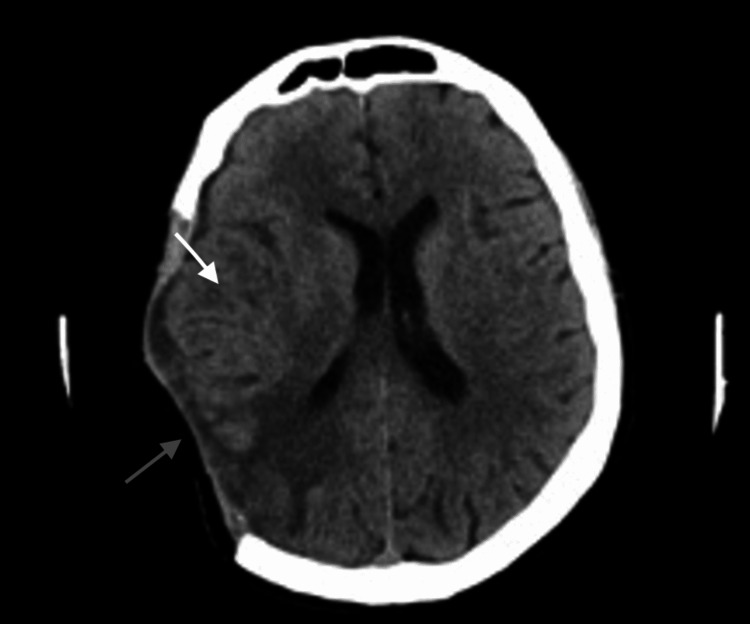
Computed tomography angiogram scan of the head showing postoperative changes (gray arrow) with right hemispheric edema similar to prior studies (white arrow)
